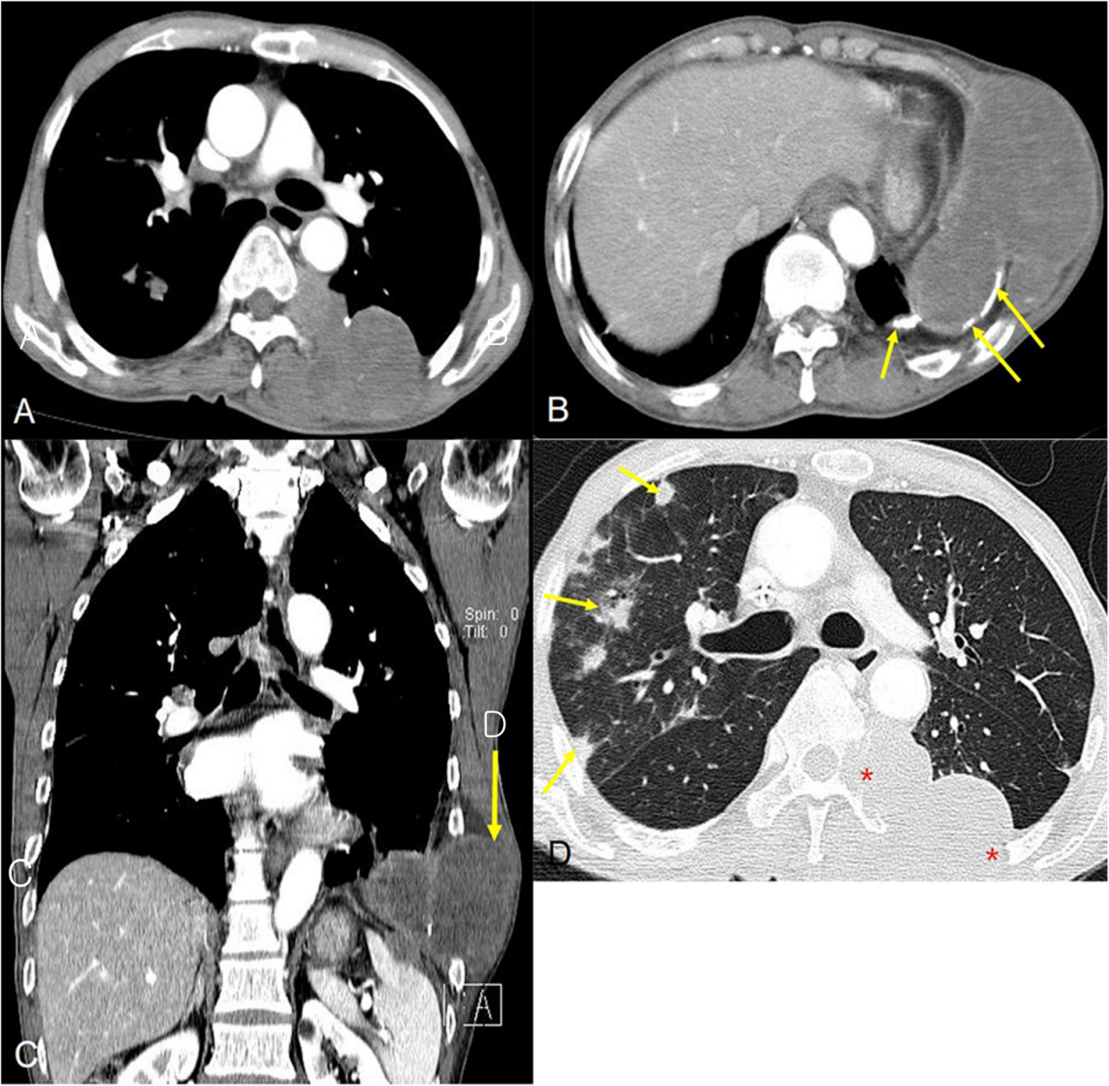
Primary pleural angiosarcoma in a 75-year-old man with a history of tuberculosis. Enhanced multidetector CT scan (A, B) showed two, sharply demarcated, biconvex, hypodense masses, arising from chronic tuberculous empyema sequelae with calcific plaques. These masses were directly invaded the extrathoracic regions, resulting in adjacent left 6-8^th^ ribs destruction (B, C). Lung scan (D) CT showed multiple hematogenous metastases in both lung fields, and left rib posterior arc change
